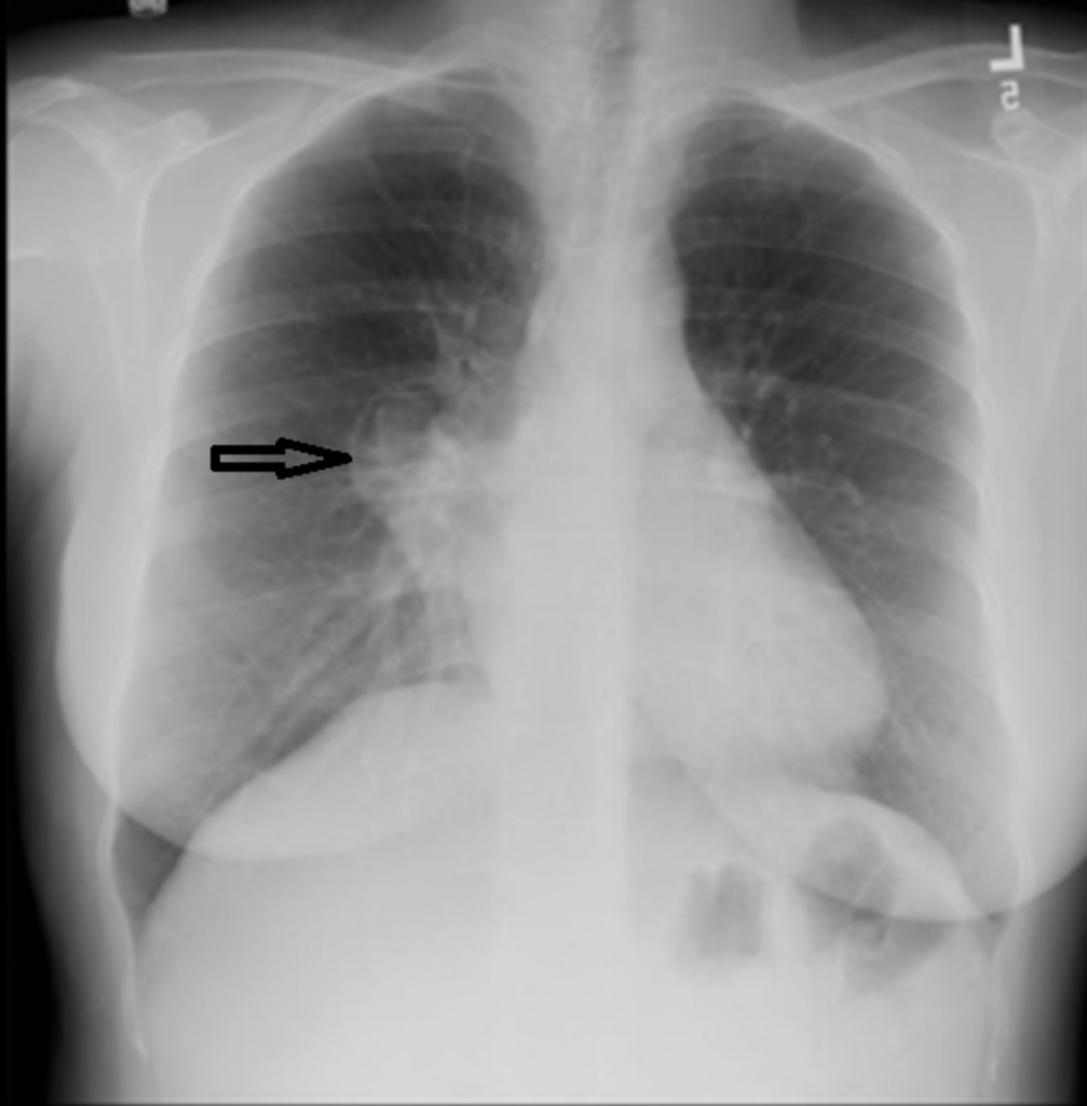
Chest X-ray revealed a right-sided para-pericardial mass in the anterior mediastinum and a significant decrease in the size of the right middle lobe opacity
